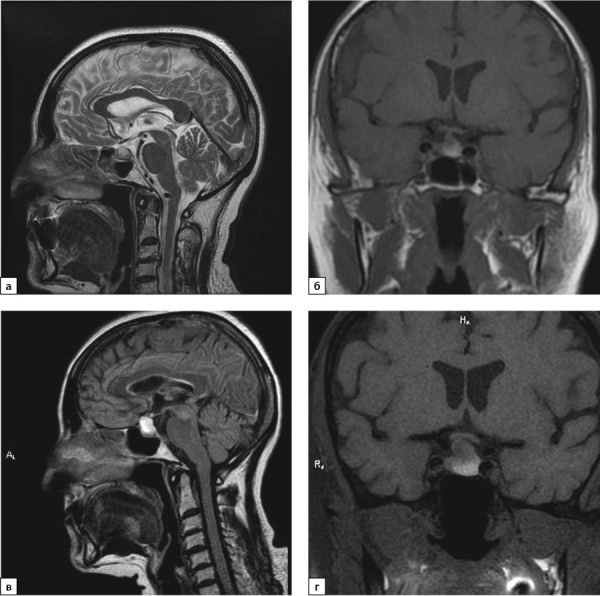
Рисунок 1. МРТ от 2019 г.: а) Т2-ВИ, сагиттальный срез; б) Т1-ВИ, корональный срез. МРТ от 2021 г.: в) Т2 FLAIR-ВИ, сагиттальный срез; г) Т1-ВИ, корональный срез.Примечание. ВИ — взвешенное изображение.Figure 1. MRI from 2019: a) T2-WI, sagittal section; b) T1-WI, coronal section. MRI from 2021: c) T2 FLAIR-VI, sagittal section; d) T1-WI, coronal section
